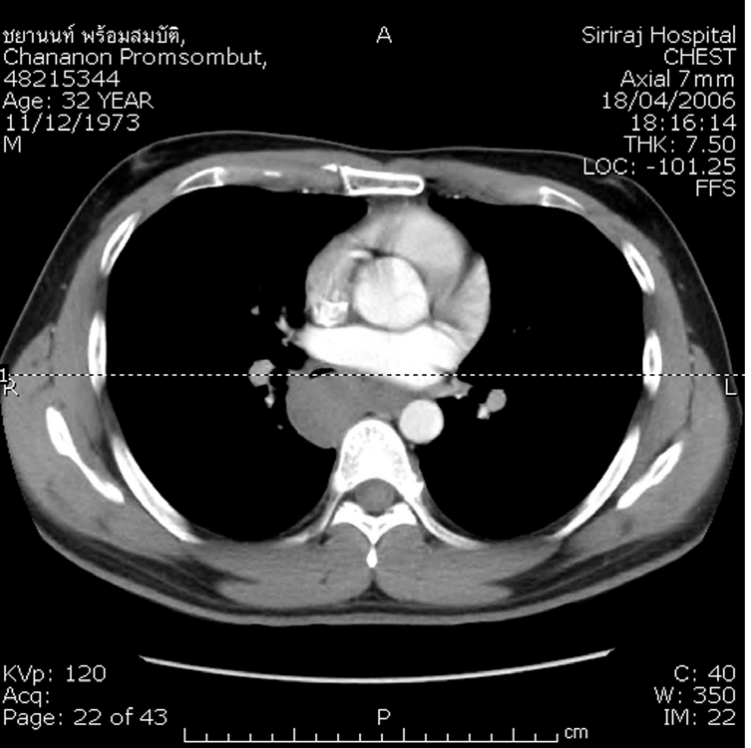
Computed tomography scan of the chest showing the esophageal tumor mass bulging toward the right pleural cavity.
